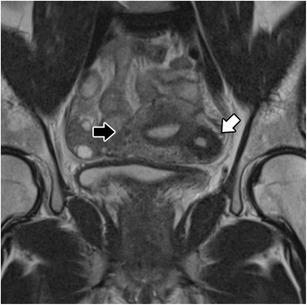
Isolated or juvenile cystic adenomyoma: Coronal T2-weighted images; nodular uterine lesion with a central cavity with hyperintense signal (white arrow), without connection to the endometrial cavity in an otherwise normal uterus (black arrow)
